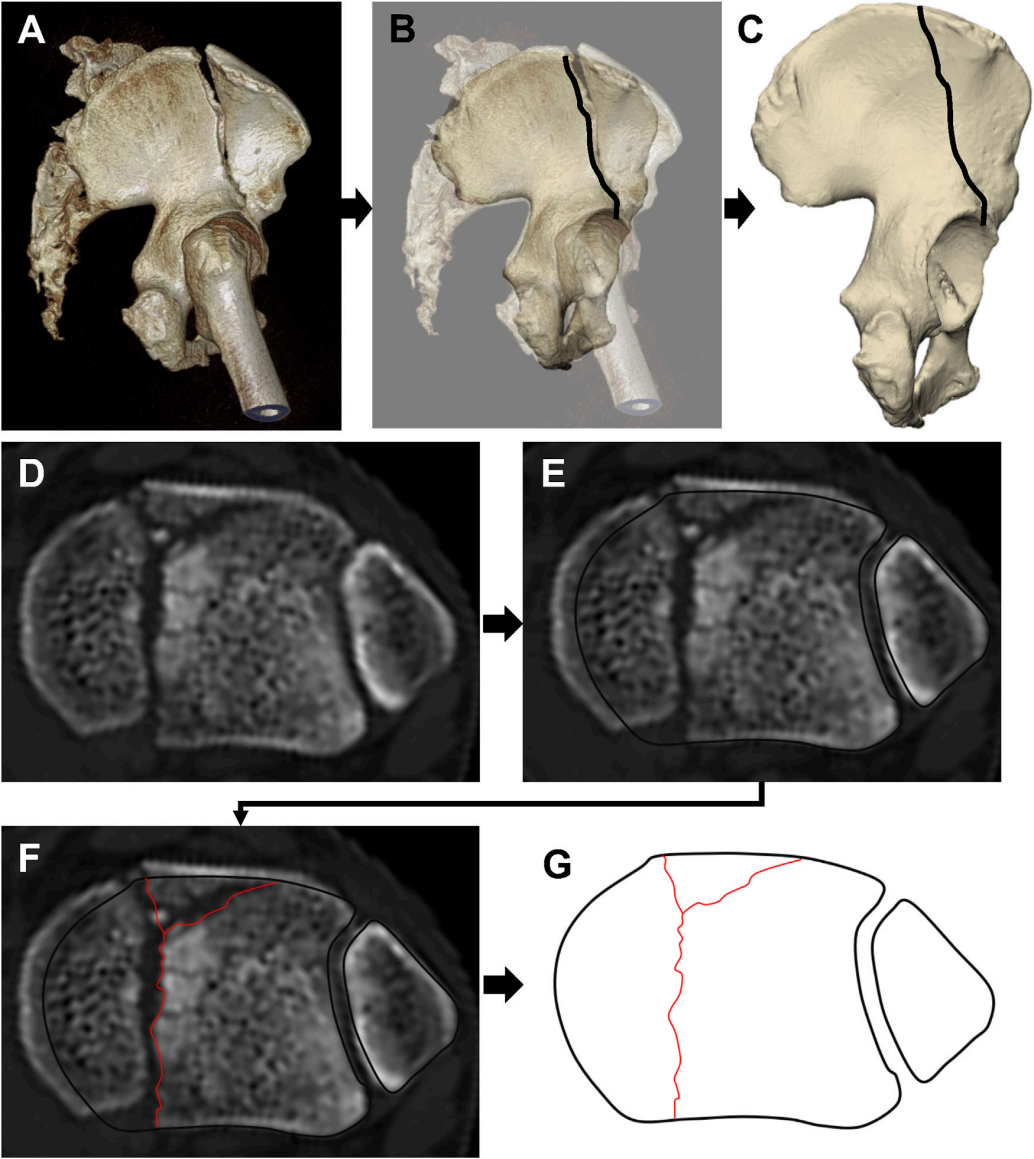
A series of images showing the process of 2D fracture mapping, starting with (A) a computed tomography image, (B) mapping the fracture line onto a matching standard template, and (C) ending with the fracture line mapped onto the standard template. (D–G) Superimposition of CT transverse images for fracture mapping: (D) CT cross-sectional image 3 mm below the unreduced tibial articular surface; (E) Alignment of the CT image with the template; (F) Fracture line transcription onto the standard template; (G) Completed fracture line transcription.
